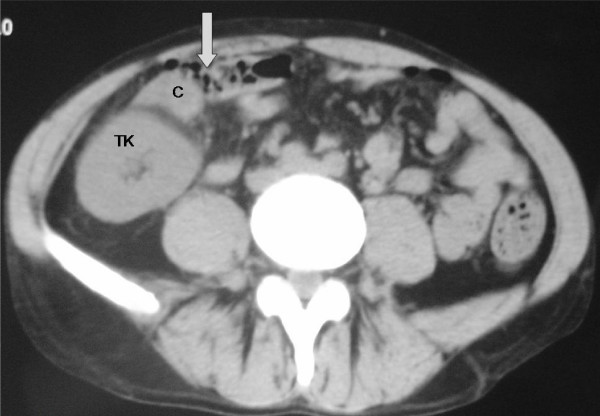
Intra-abdominal free air identified on abdominal computed tomography scan.
